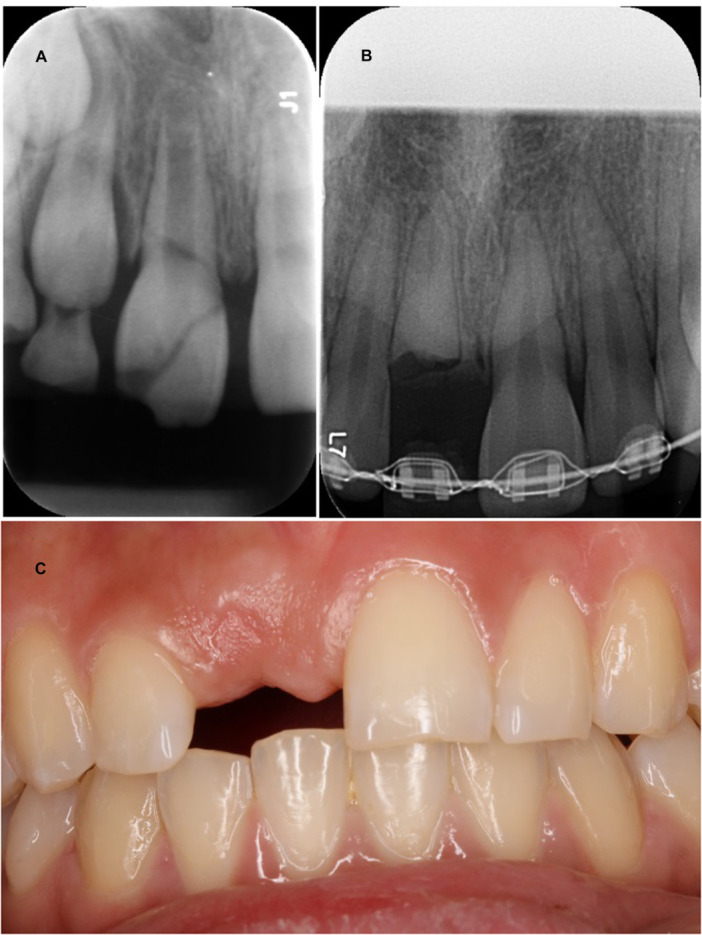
(A–C) Patient case: 11 were diagnosed with crown‐root fracture with pulpal involvement when the patient was 8 years old. (A) Radiograph from the day of the injury before initial treatment. Due to the severity of the fracture, the tooth could not be restored. The patient was referred to the Regional Dental Care, Department of Oral and Maxillofacial Surgery, Copenhagen University Hospital. To prevent resorption of the labial bone in the anterior maxilla, decoronation of 11 was performed. Following consultation with the interdisciplinary team, the treatment plan identified a dental implant as the most appropriate permanent solution. This required more space to be created in region 11. Additional space was created through orthodontic treatment. (B) Radiograph taken at the 8‐year follow‐up. 11 is seen decoronated, and the patient is undergoing orthodontic treatment with fixed equipment. (C) Clinical photo taken at the 12‐year follow‐up performed at the regional dental care. The patient is now 20 years old and has a denture. Due to continuous facial skeleton growth, the patient is still waiting for the permanent tooth replacement—in this case, an implant.
